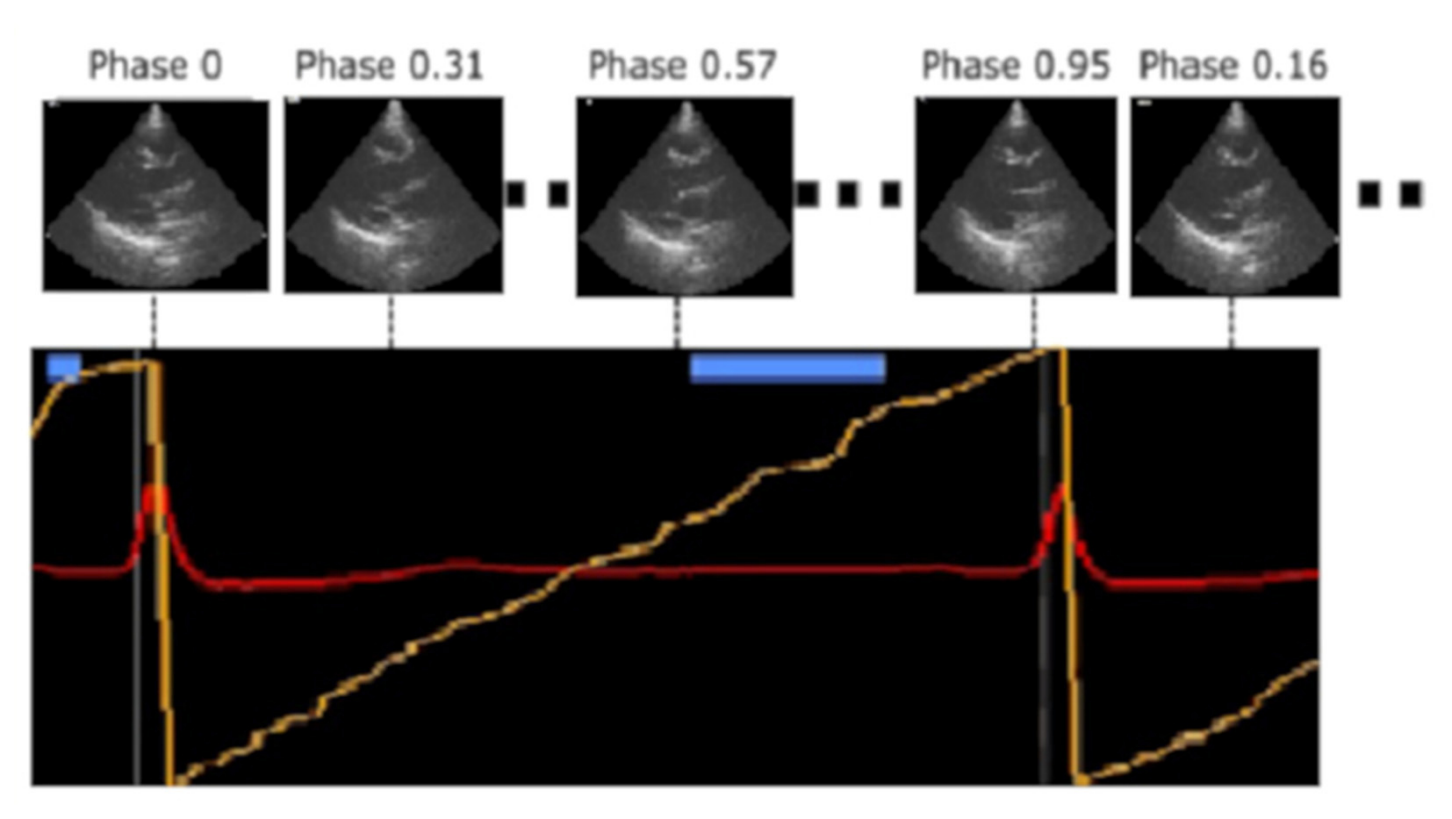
Identification of the cardiac cycle phase performed by the artificial intelligence algorithm through the real-time analysis of the acquired ultrasound images. A linear mapping between 0 and 1 (yellow line) was performed in the R-R peak interval and a cardiac phase was assigned to each ultrasound frame based on its temporal position within this interval.
